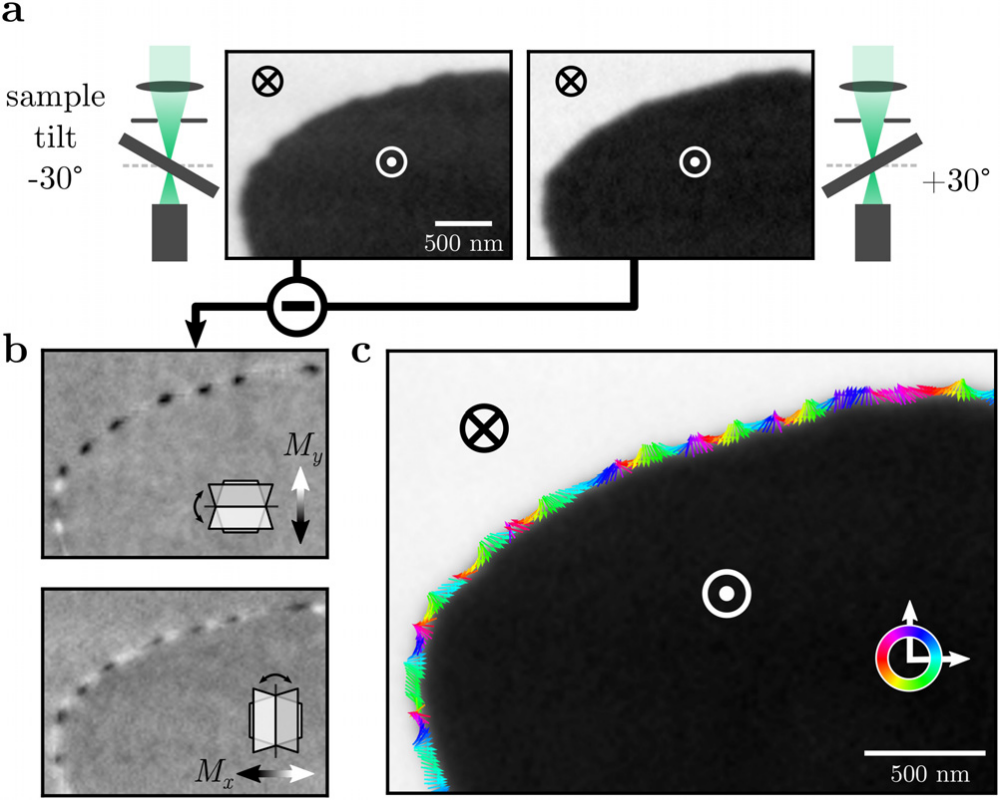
XMCD vector imaging and reconstruction. (a) STXM-images of a domain wall section, recorded with circular polarized x-rays for
